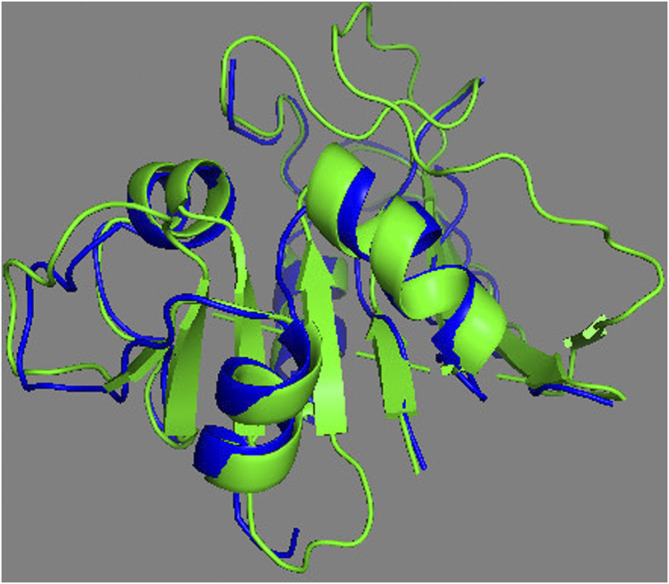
Superposition of the calculated fragments by REDCRAFT (blue) and the X-ray structure of DHFR (green).
